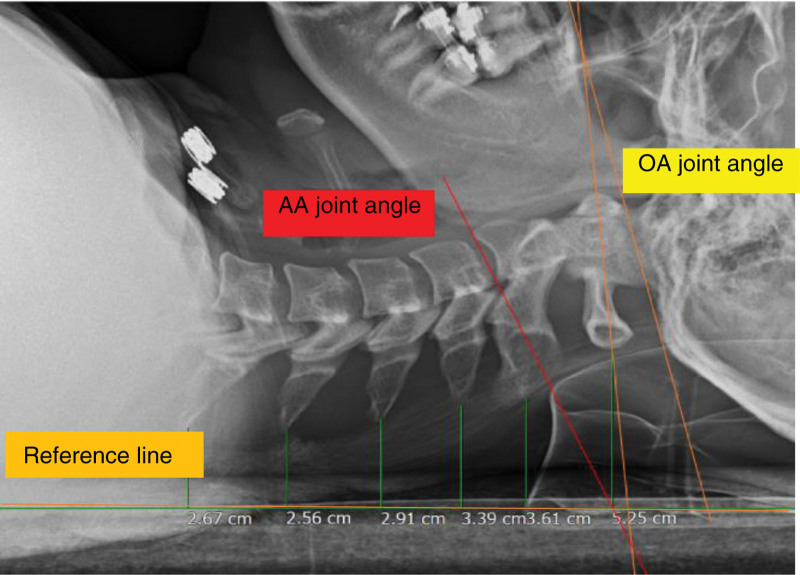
Measurement of the OA and AA joint angles and the CSPPD for C1–C6 during low stage CCFT (20–24 mm Hg) using radiography in a participant with a cervical lordotic angle of more than −4°. AA = atlantoaxial, CCFT = craniocervical flexion test, CSPPD = cervical spinous process posterior displacement, OA = occipito-atlantal.
